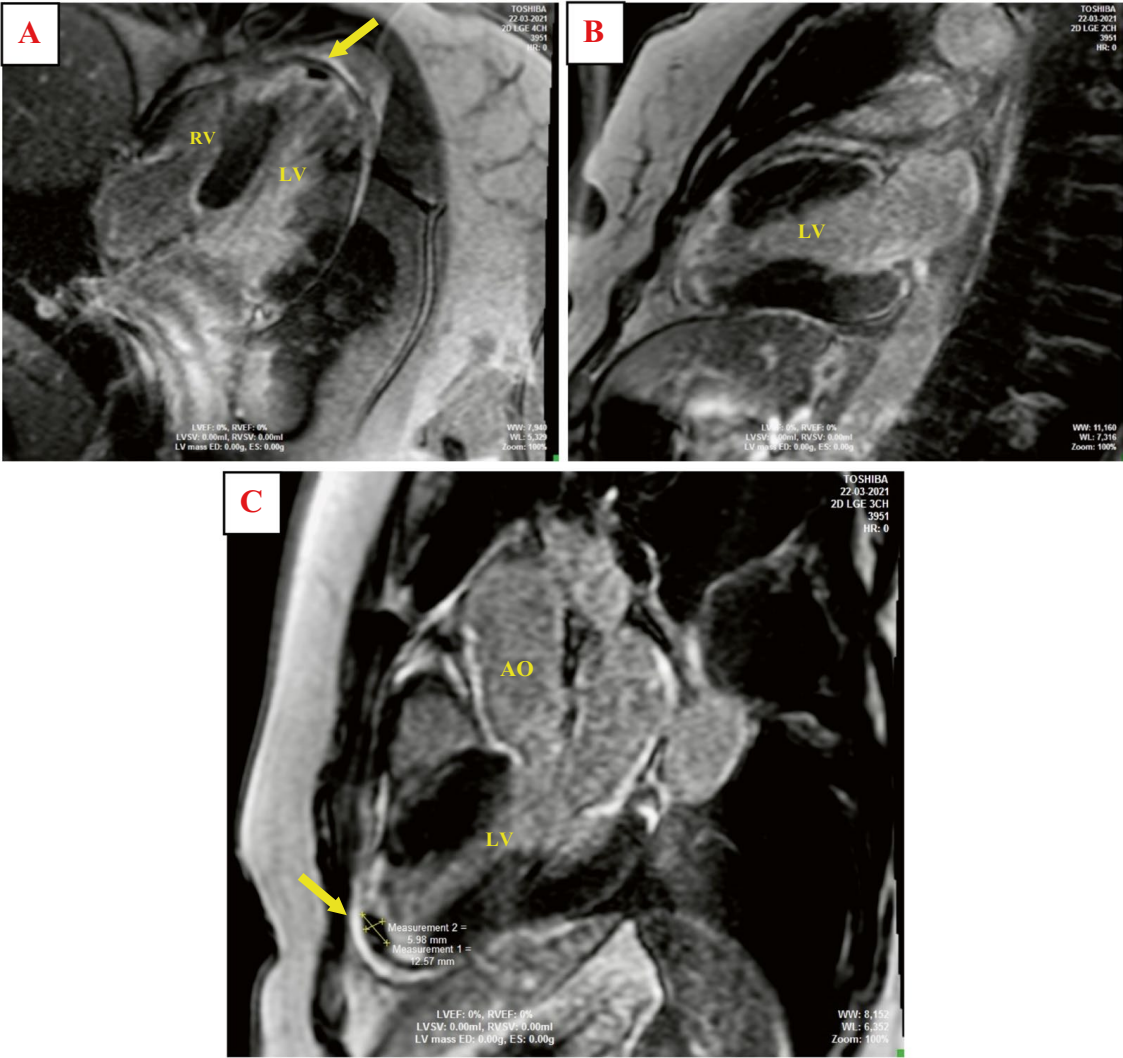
Cardiac magnetic resonance imaging (MRI) sequences of late enhancement in a A four-chamber left view and B two-chamber left view showing enhancement of the apex and all apical segments of left ventricle walls. C MRI sequences of late enhancement in a three-chamber view showing the absence of late gadolinium enhancement of an apical left ventricle mass attesting the diagnosis of intracardiac thrombus measuring 12 mm × 6 mm (yellow arrow); *RV* right ventricle, *LV* left ventricle, *AO* aorta
